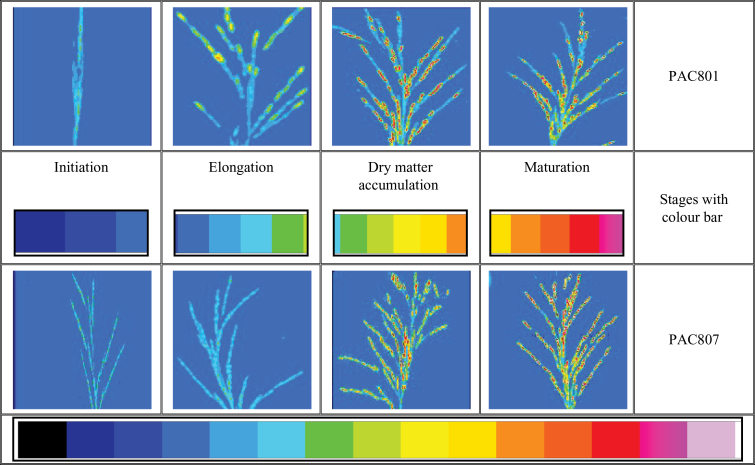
Pseudo-colouring effect on the 8-bit binary CT scan images of PAC801 and PAC807 with developmental stages by using ImageJ software. Changes from the blue to the red scales indicate changes in water content and dry matter at the different stages of seed development. (The stronger the blue colour, the higher the seed water content; the stronger the red colour, the higher the dry matter accumulation in the seed.)
